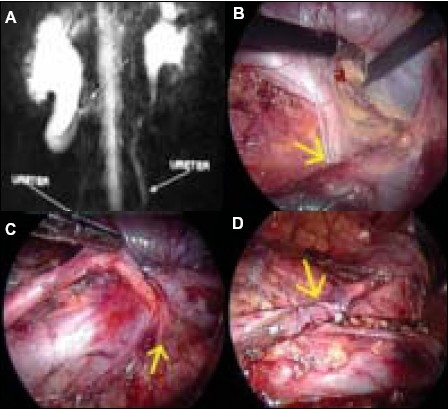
A. Showing MRI of retrocaval ureter showing “fish hook” deformity, B. Dilated proximal ureter dissected out upto the lateral border of IVC. C. Distal ureter a mobilized in the interaortocaval region. D. Ureteroureterostomy done with 4.0 vicryl by intracorporeal suturing.
